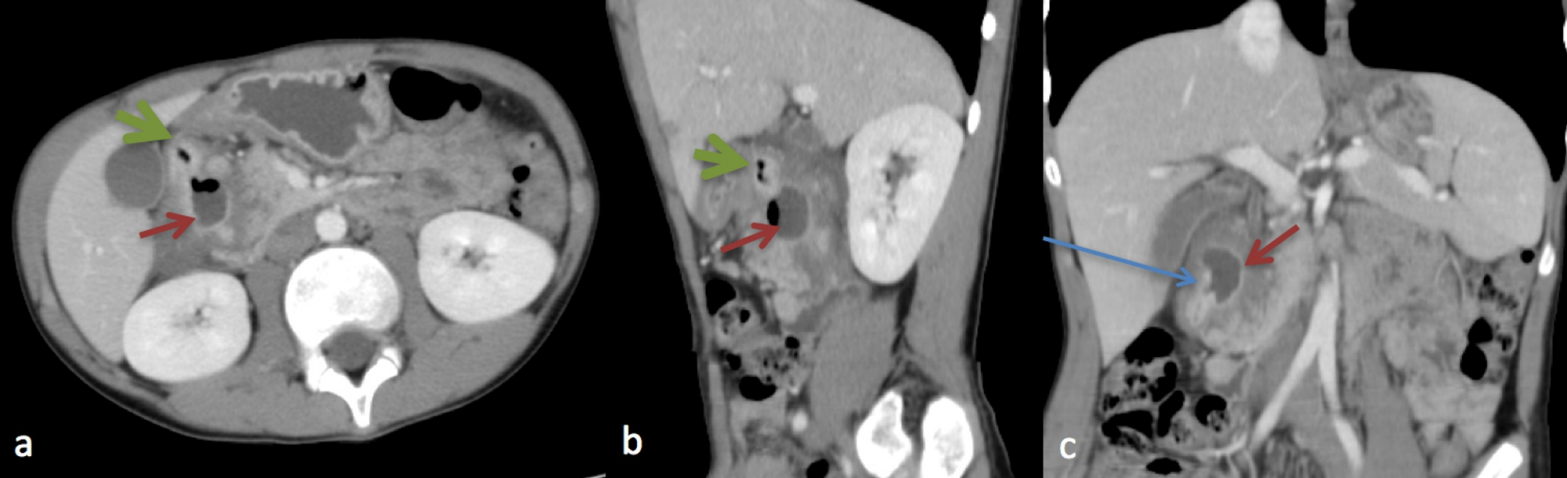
Selected contrast enhanced CT scan of the abdomen in axial (a), with sagittal (b) and coronal (c) reconstructed images, demonstrates the duodenal diverticuli (green and red arrows) outpouching from the second duodenum, medial in location. The larger diverticulum shows air fluid level. The diverticular neck is better depicted in the coronal plane (blue arrow). Noted are the periduodenal inflammatory changes with bowel wall thickening and edema.
